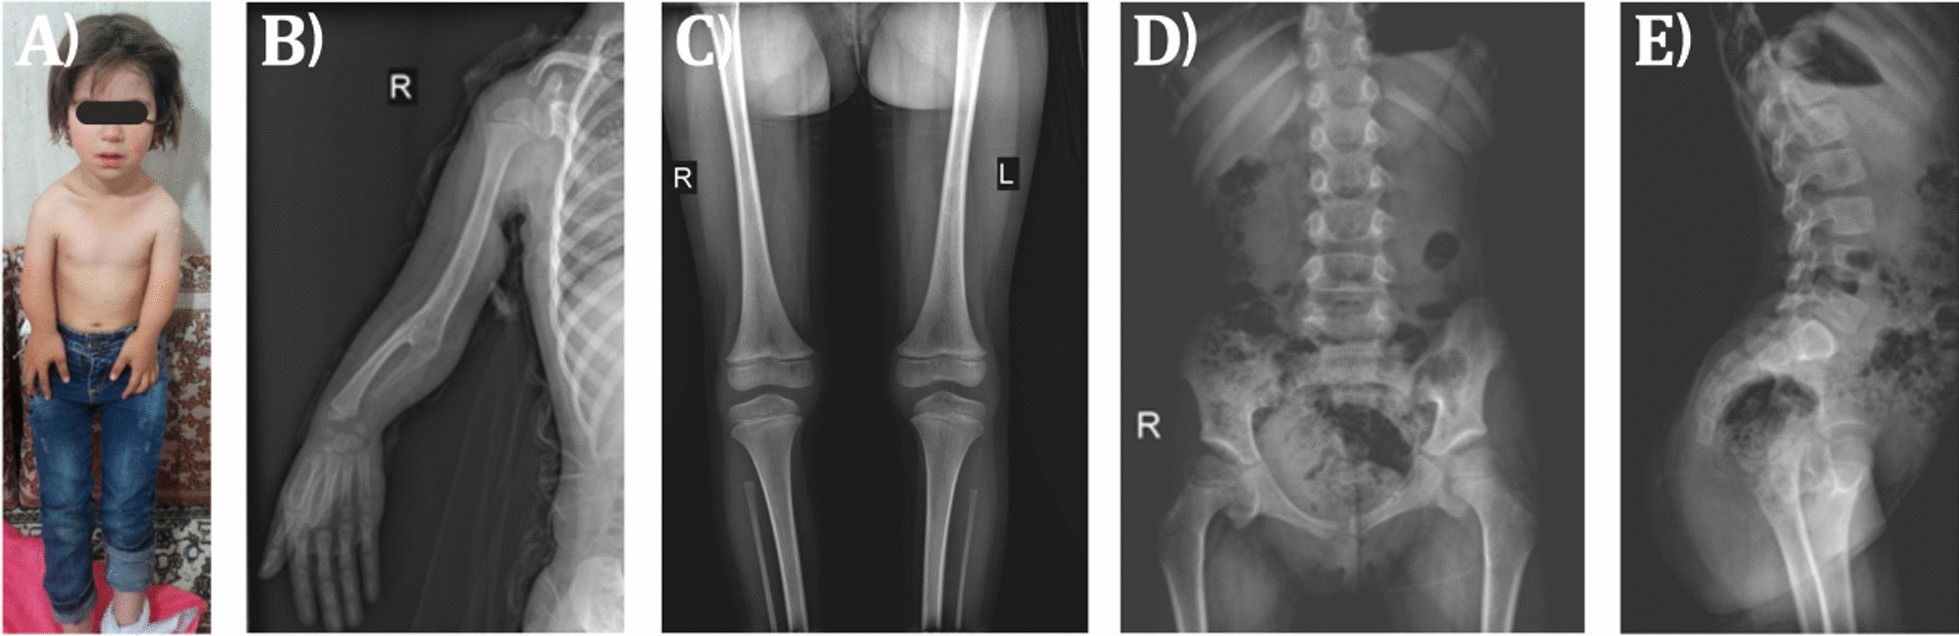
A Short stature and mesomelic shortening of arms in the patient. B–E Skeletal X-ray surveys of the patient's right upper extremity (B), lower extremities (C), anterior view of the lower spine and pelvis (D), and lateral view of the lower spine and pelvis (E)
